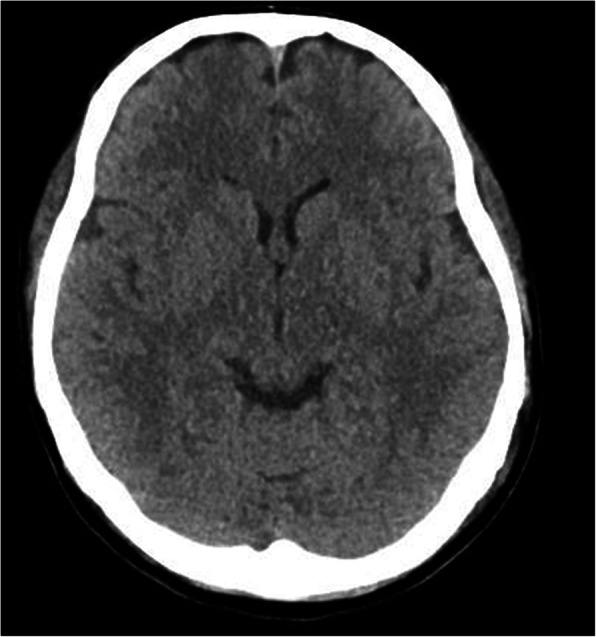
Head computed tomography immediately after the seizure attack. The image demonstrates mild cerebral edema 7 h after ICU admission
